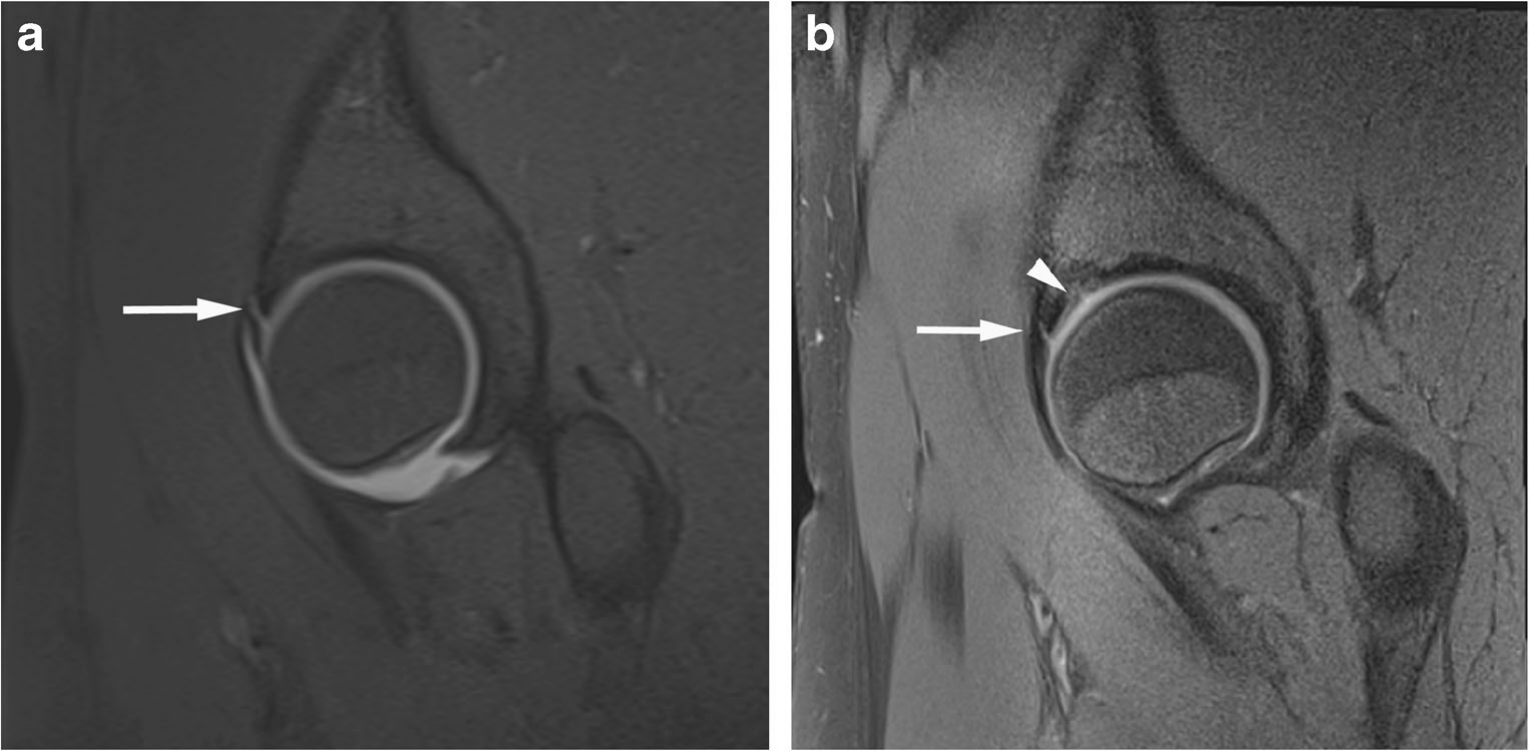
Anterosuperior acetabular labrum complete tear with adjacent full thickness cartilage defect in a 26-year-old confirmed at surgery. Sagittal (a) T1 FS 1.5T MRA shows complete basal labral tear (arrow) and normal articular cartilage and (b) PD FS 3T MR image shows complete basal labral tear (arrow) and full thickness cartilage defect (arrowhead)
